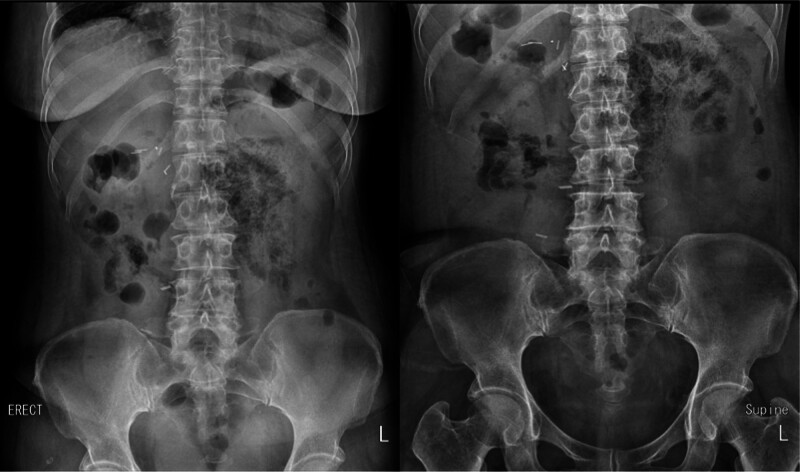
Plain X-ray of the abdomen (erect and supine) performed on the fifth day of hospitalization.
